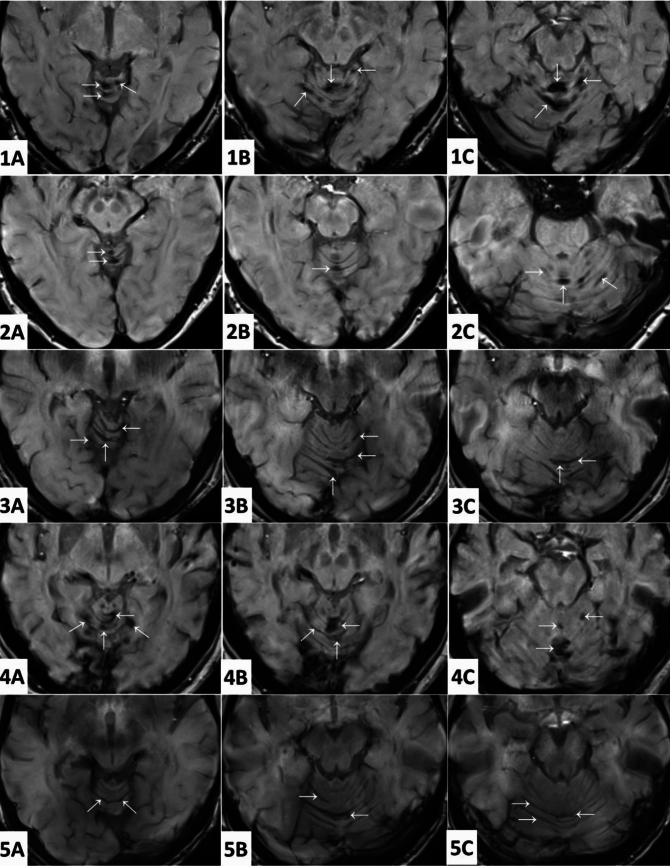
Axial susceptibility-weighted magnetic resonance images, with typical findings suggestive of iSS. (Cases 1–5). Cephalad to caudal appearances (A–C) of superior vermis and cerebellar folia. Hypointense signal changes suggestive of hemosiderin deposits are marked with arrows. Source: UK Biobank ©. Reproduced by kind permission of UK Biobank ©
